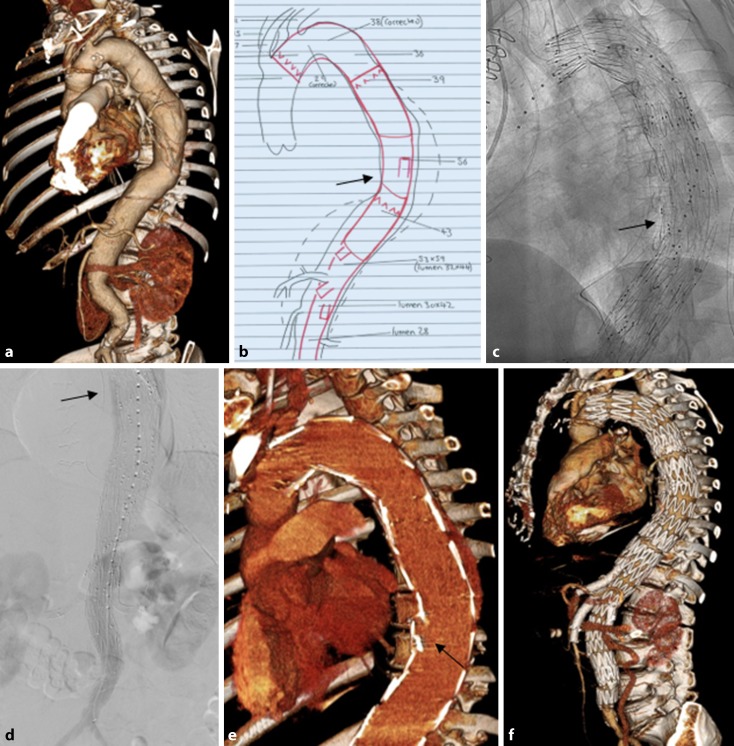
A female patient with a thoracoabdominal aortic aneurysm (a) treated using a four-vessel branched stent graft with a perfusion branch (*arrow *in b). The perfusion branch (*arrow*) following graft placement (*c*) and aneurysm perfusion (*arrow*) on late final angiography (d). Follow-up computed tomography angiography shows the open perfusion branch (*arrow* in e) and complete repair (f)
